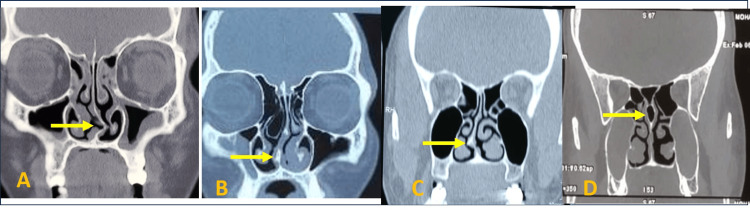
Coronal CT images of paranasal air sinuses showing nasal septal variations A: left-side deviation; B: S shape; C: spur; D: pneumatization CT: computed tomography
